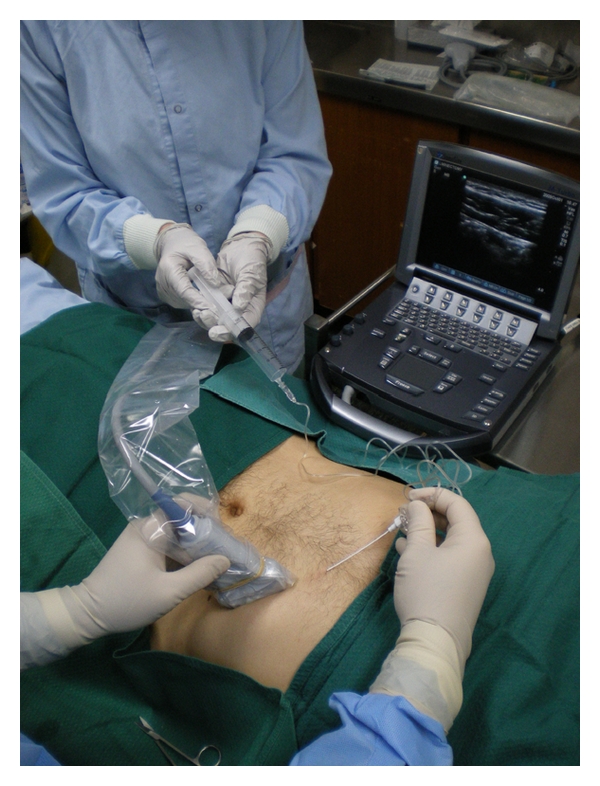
Placement of ultrasound probe for subcostal TAP blocks. Generously shared from the personal files of Prof. P. Hebbard.
